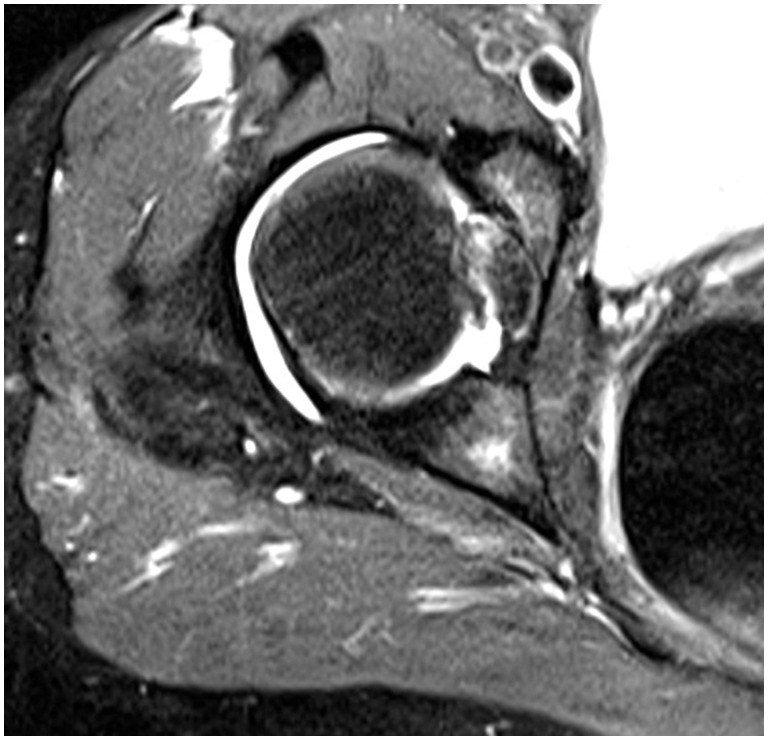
Appearance of a tear of LT on MRI.
